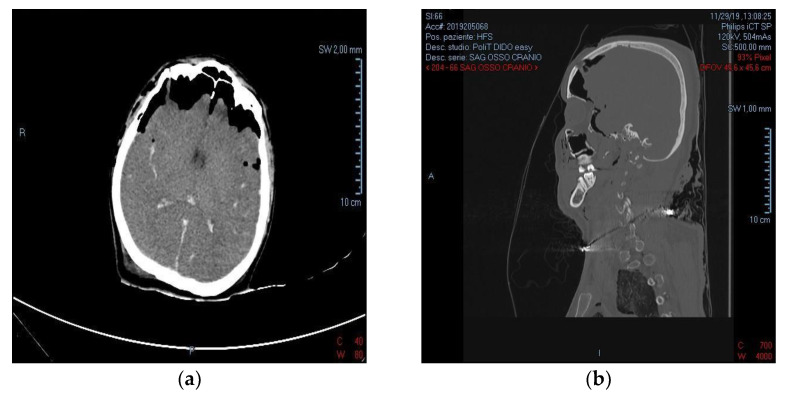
CT scan frames of the victim. (a) Transverse plane showing pneumocephalus and hemorrhages; (b) Sagittal plane showing the penetrating lesion of the skull.
